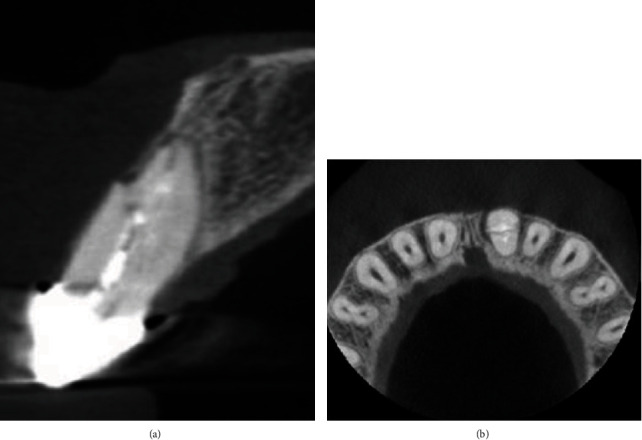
(a) After 6 months of follow-up on CBCT (axial view). (b) After 6 months on CBCT (sagittal view).
